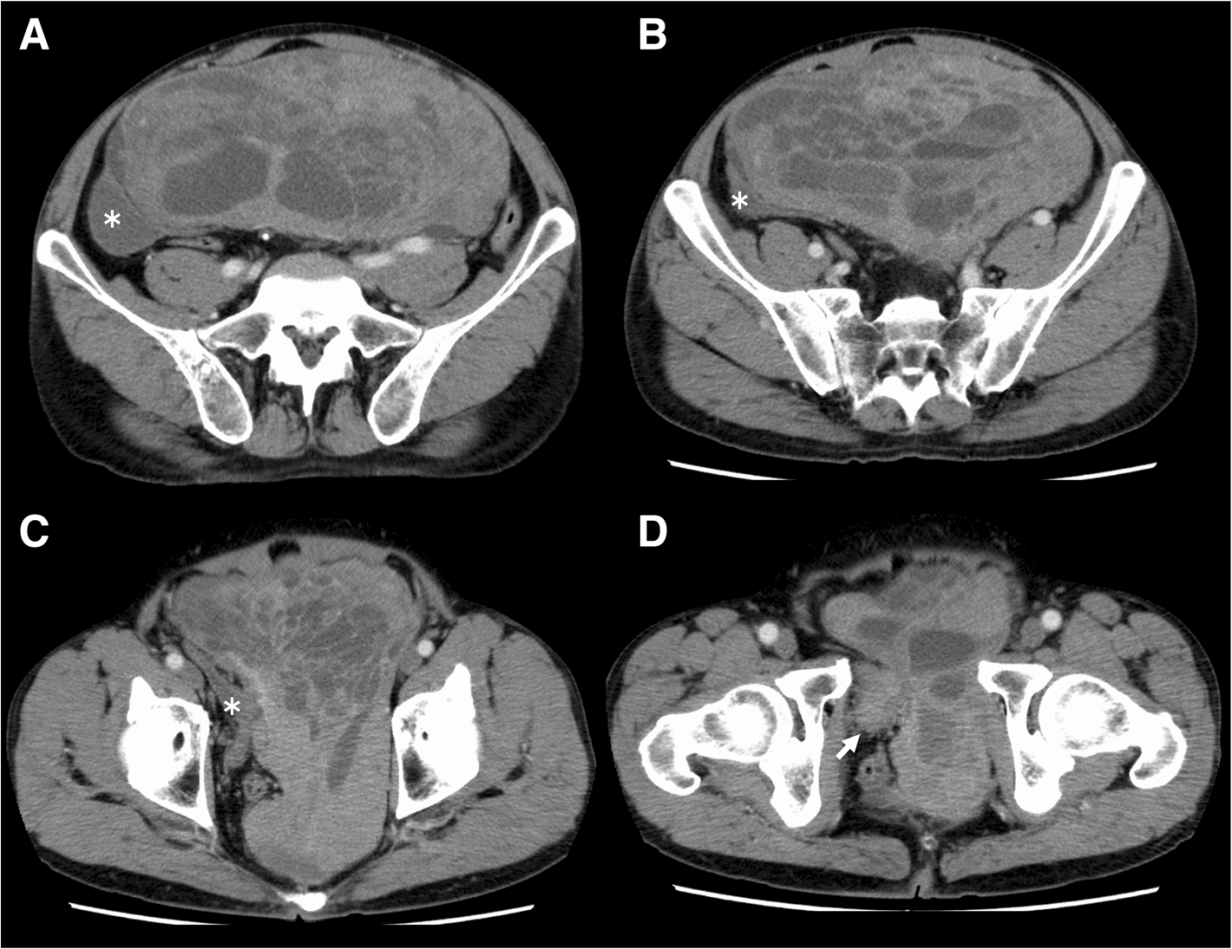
Enhanced abdominal CT. a, b, c A large multilocular mass with soft tissue component displacing the bladder (*) and rectum anterolaterally. d The arrow shows the prostate
